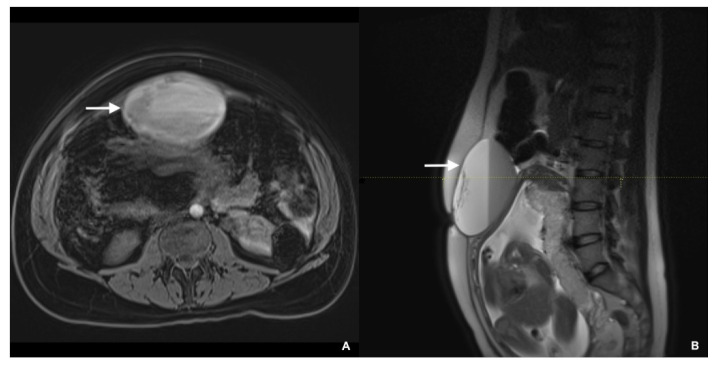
(A) Abdominal MRI axial T1-weighted spoiled gradient-echo post-Gd administration image showing a multiloculated anterior swelling of the uterine fundus, with a partially hematic liquid content. (B) Abdominal MRI sagittal T2-weighted image showing the mass extending cranio-caudally and the fetus in the gravid uterus.
